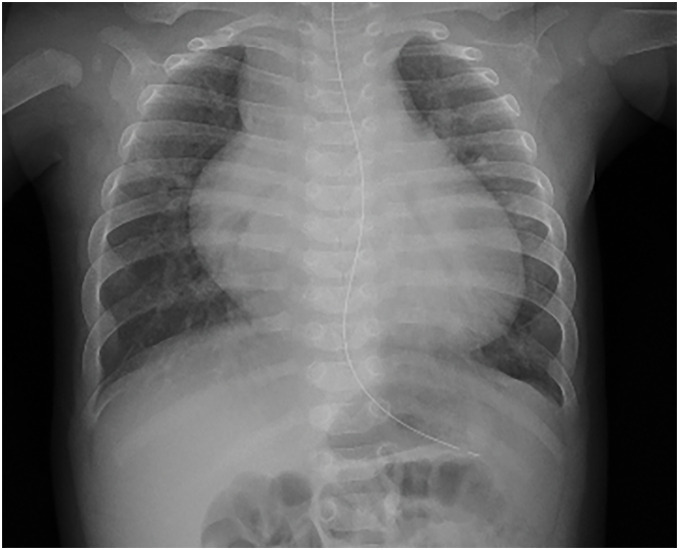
Preoperative chest radiograph of patient 1. There is significant cardiomegaly.
